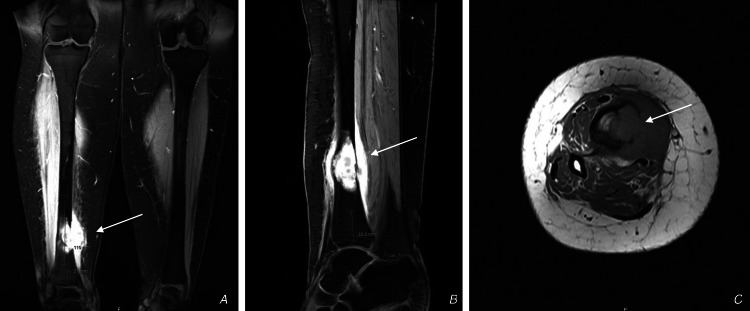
MRI images at the time of admission A: Shows a coronal cut of both tibias, B: Shows sagittal cut of the right distal tibia, C: Shows an axial cut of the right distal tibia. Arrows show the tumor invading the anterior and posterior deep muscle compartments of the right leg.
